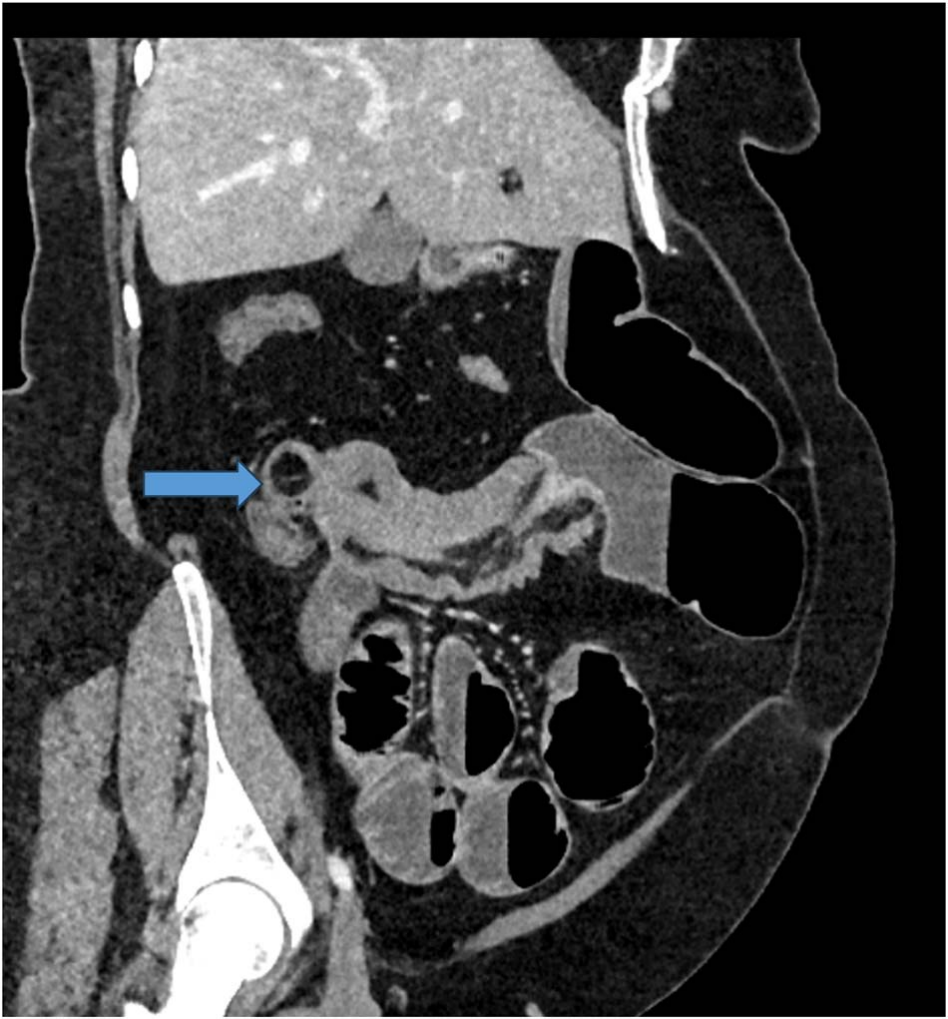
CT scan of abdomen: oblique coronal view of abdomen showing intussusception with low attenuation lesion distally C/W a lipoma (arrow).
